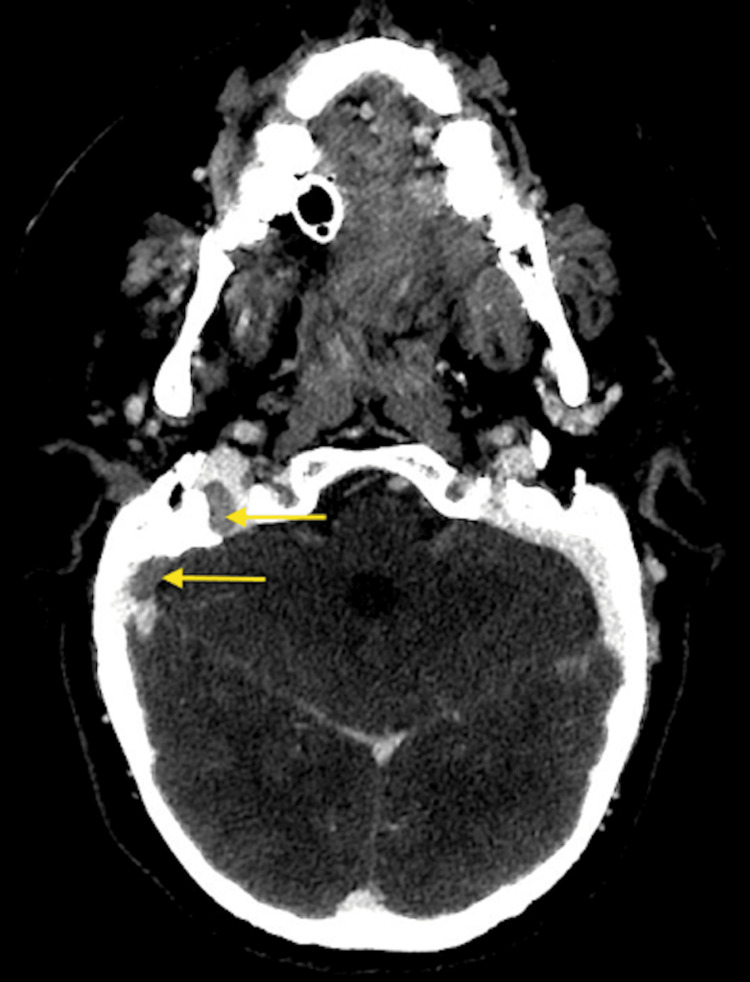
Cerebral CT Venography (axial) demonstrates an extensive filling defect within the distal right transverse sinus extending into the right sigmoid sinus and jugular bulb (arrows), consistent with non-occlusive DVST. DVST: dural venous sinus thrombosis
